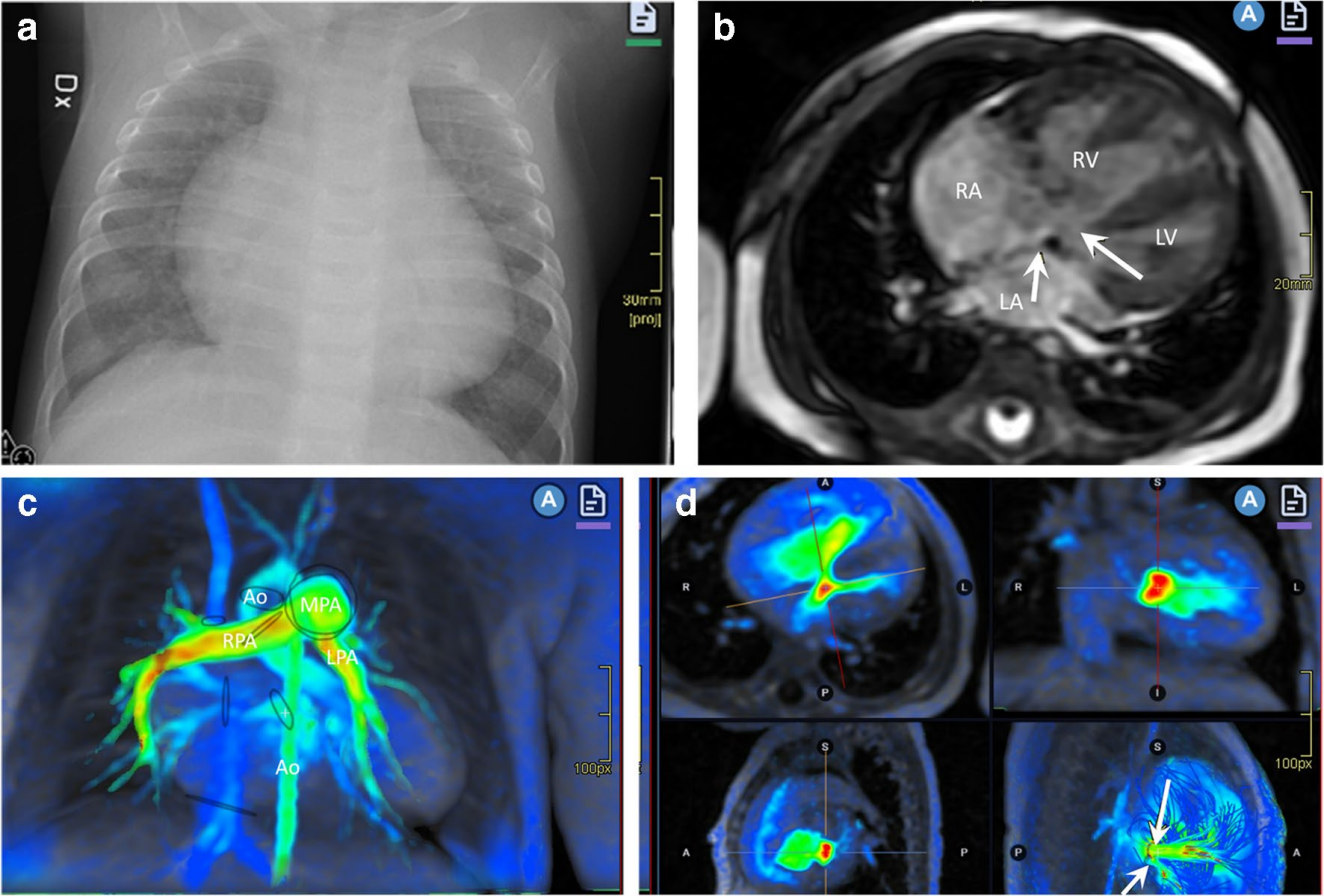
A 7-month-old girl presenting with acute cardiac failure due to a previous undiagnosed incomplete atrioventricular septal defect with mitral stenosis and a small left ventricle. a Anteroposterior chest radiograph shows an enlarged heart with congestive pulmonary vessels. b Magnetic resonance 2-dimensional (D) steady state free precession image in a 4-chamber view reveals an enlarged right ventricle and right atrium, the atrial primum defect (*short arrow*) and ventricular septal defect (*long arrow*). c, d A 4-D phase contrast flow sequence (4-D flow) where the color coding represents blood flow velocity; from low velocity in blue to high velocity in red. c A volume rendered image with traced regions of interest on the different vessels, valves, and shunts showing an enlarged main pulmonary artery and branches. d Multiplanar reconstruction with regions of interest marked on the septal defects (*arrows*). The 4-D flow sequence enabled visualization of the mitral stenosis (*red color*) and septal shunts as well as and direct quantification of the shunts with Qp:Qs = 4:1. *Ao* aorta, *LA* left atrium, *LPA* left pulmonary artery, *LV* left ventricle, *MPA* main pulmonary artery, *RA* right atrium, *RPA* right pulmonary artery, *RV* right ventricle
